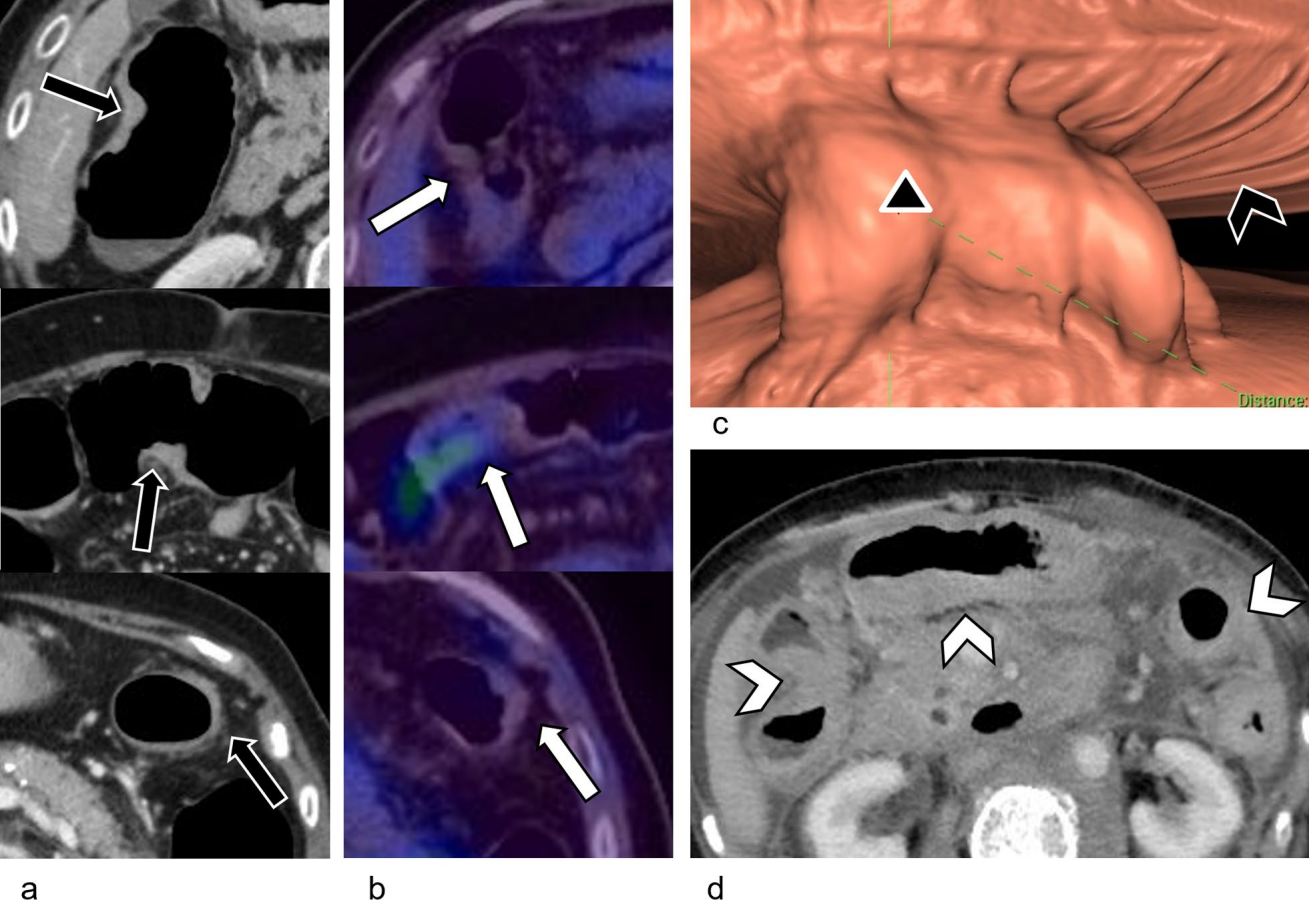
Metastatic ILC of the colon in a 77-year-old female with recurrent episodes of subacute intestinal obstruction over 6 months. She had completed therapy for Stage 3A ILC more than 10 years ago. a Axial contrast-enhanced CT colonography shows focal segments of smooth, mural thickening (black arrows) in the hepatic flexure (top), transverse colon (middle), and descending colon (bottom), mimicking peristalsis. No discrete mass is seen. b
